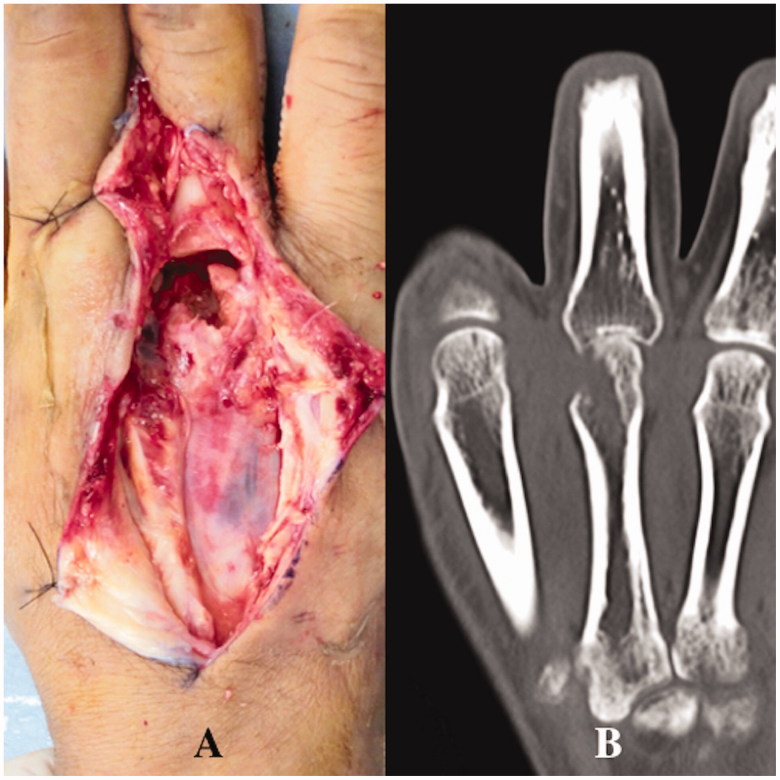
Case 2. (A) Intraoperative view showing the partial lesion of the extensor and the second metacarpal head due to bite and infection. (B) CT showing the third metacarpal head defect and the narrowed joint space.
